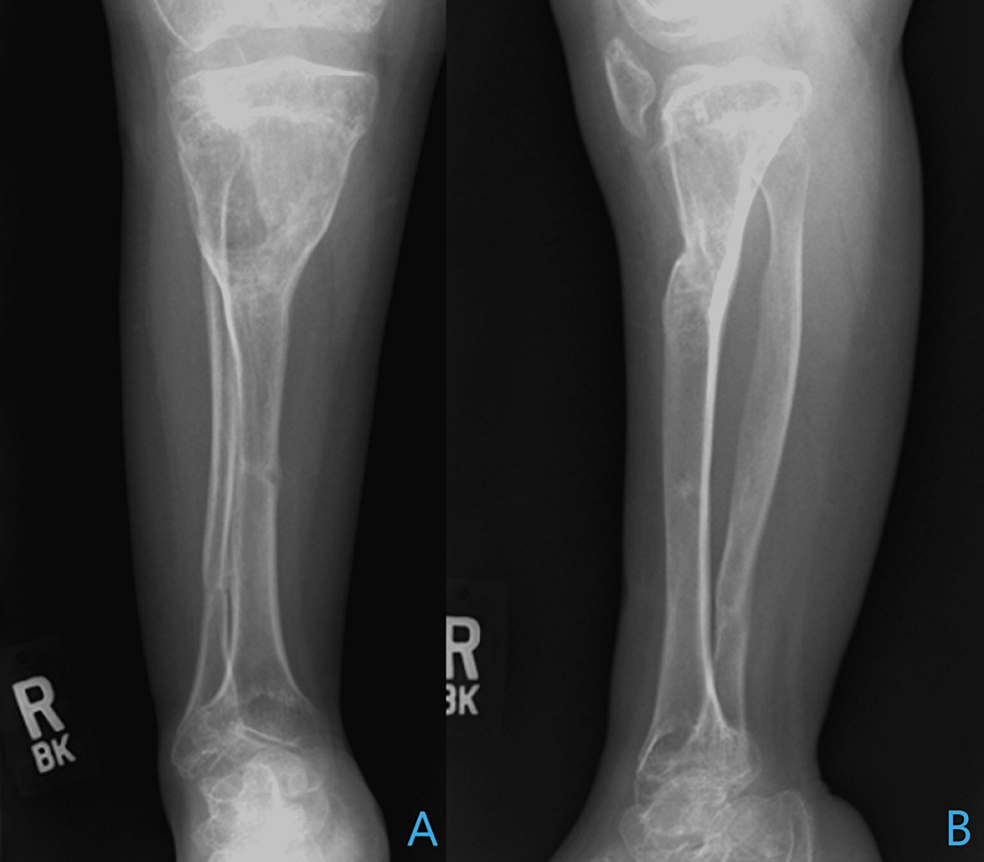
Radiography at one year follow-up Weight-bearing anteroposterior (A) and lateral (B) radiographs of the right tibia and fibula one year postoperatively demonstrate complete bony healing of the tibial and fibular osteotomy sites.
